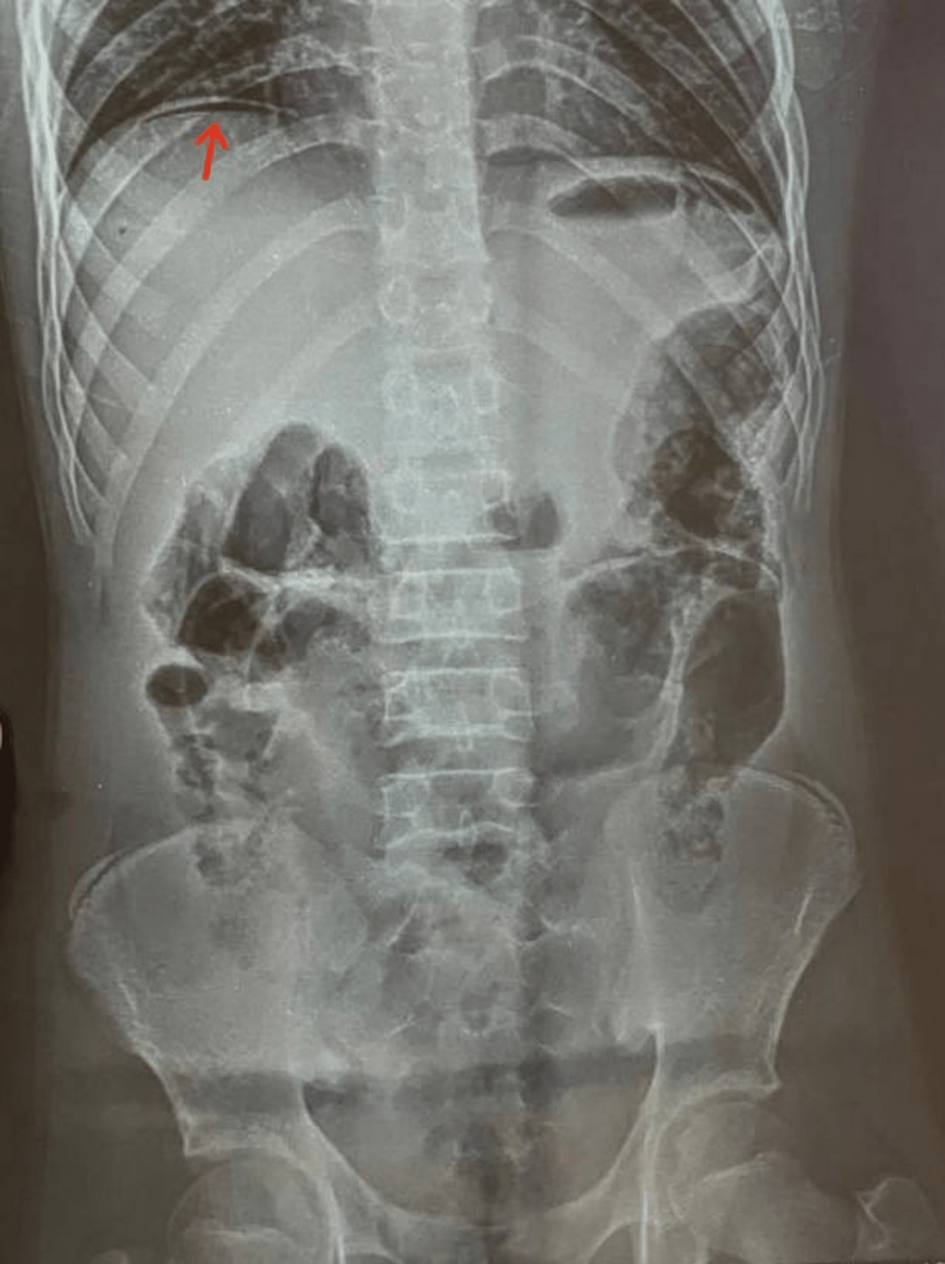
Radiograph of the abdomen in the standing position showing air under the right hemidiaphragm, as indicated by the arrow.
